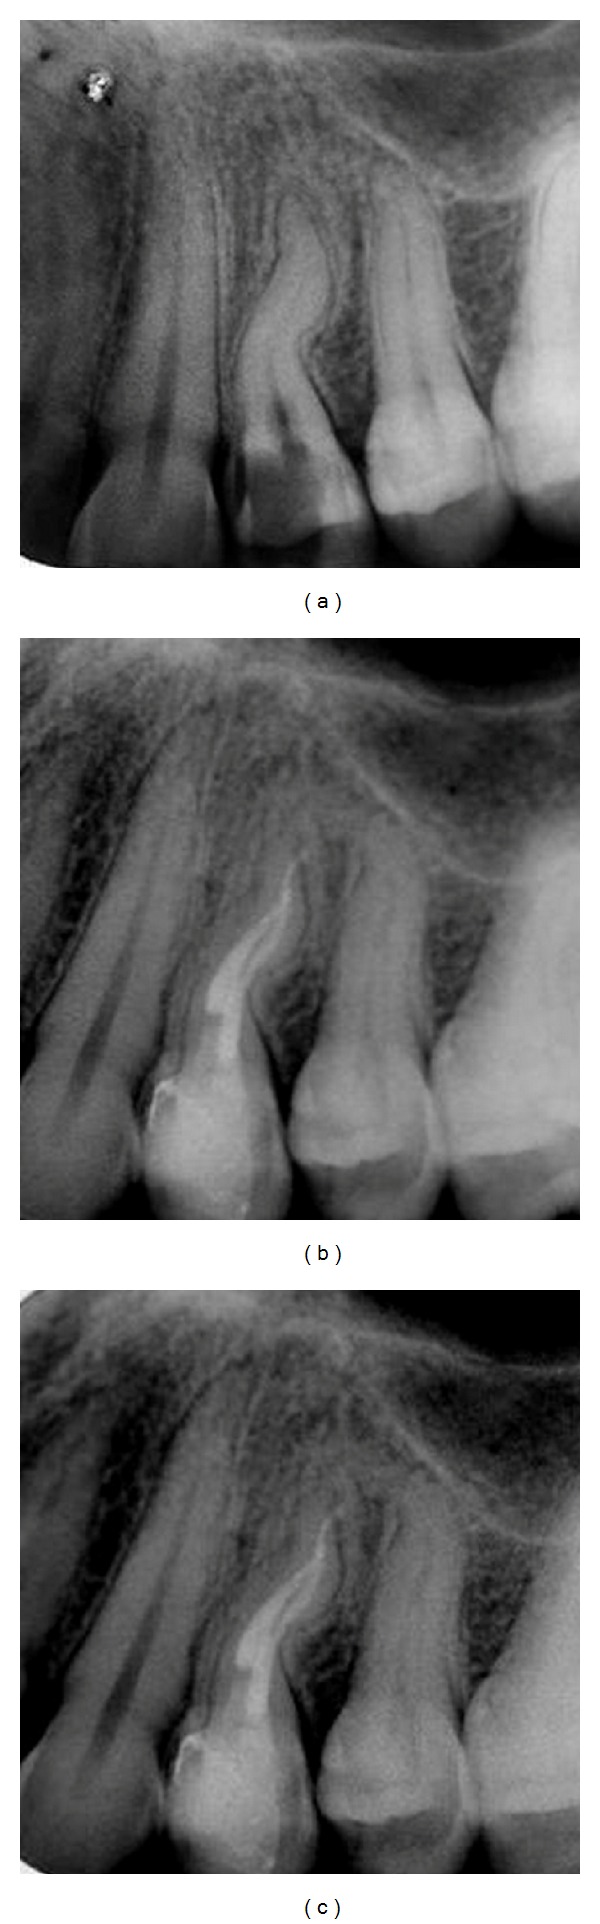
(a) Initial radiograph, (b) final periapical radiograph (straight view), and (c) final radiograph (mesial view).
